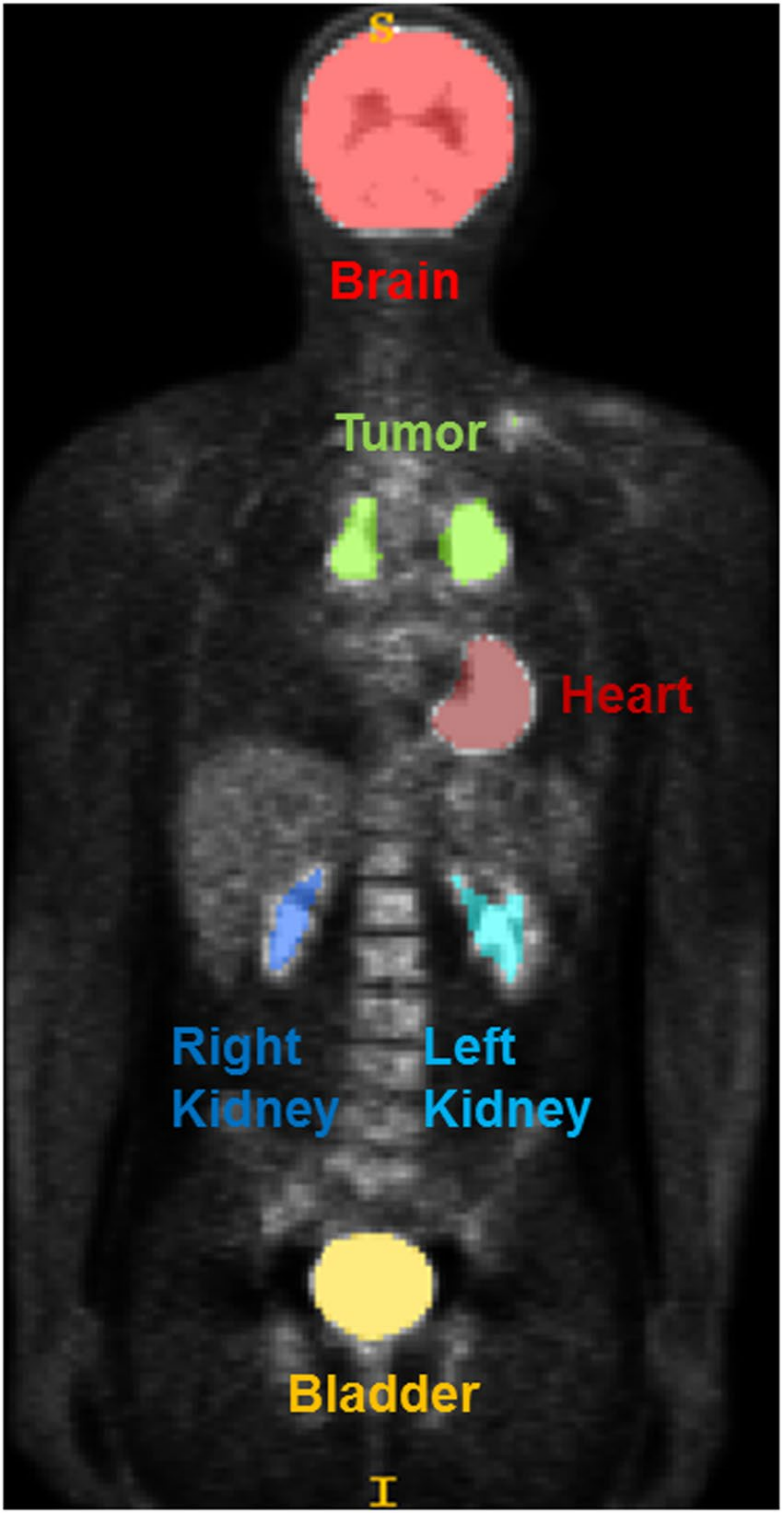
Coronal view of a FDG-PET scan from the time of diagnosis from a 15 year old male with clinical stage IIA nodular sclerosing Hodgkin disease prior to the initiation of chemotherapy with the Stanford V regimen. After image processing and SUV thresholding, FDG-PET avid tissues, such as brain (red), tumor (green), heart (dark red), right kidney (dark blue), left kidney (light blue) and bladder (yellow), are segmented for radiomics feature computation to construct random forest classifiers.
